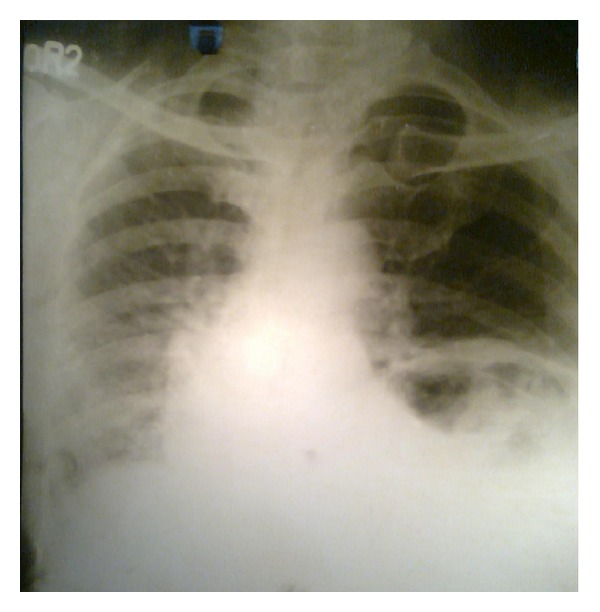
Chest X-ray PA view showing a heterogeneous opacity in the left lower zone.
